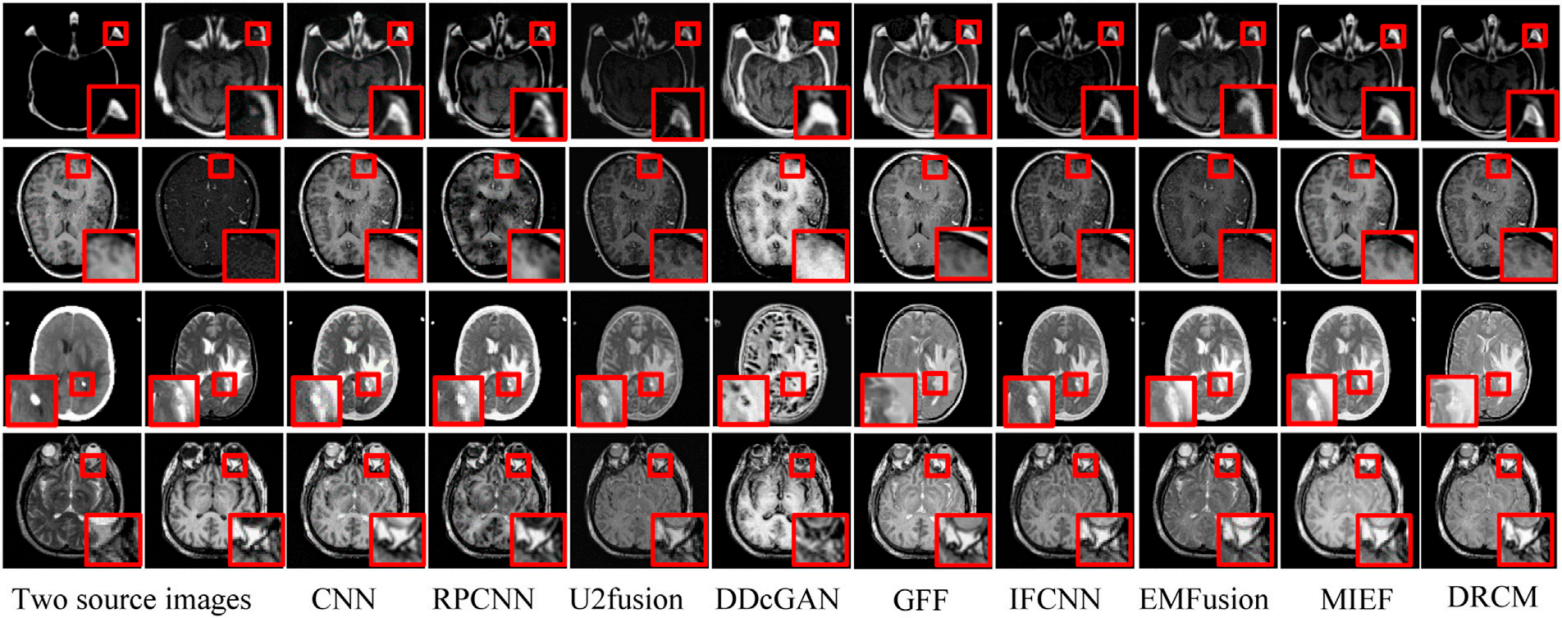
CT-MRI fusion images under different comparison methods. Two source images are reproduced with permission from http://www.med.harvard.edu/AANLIB/home.html.
